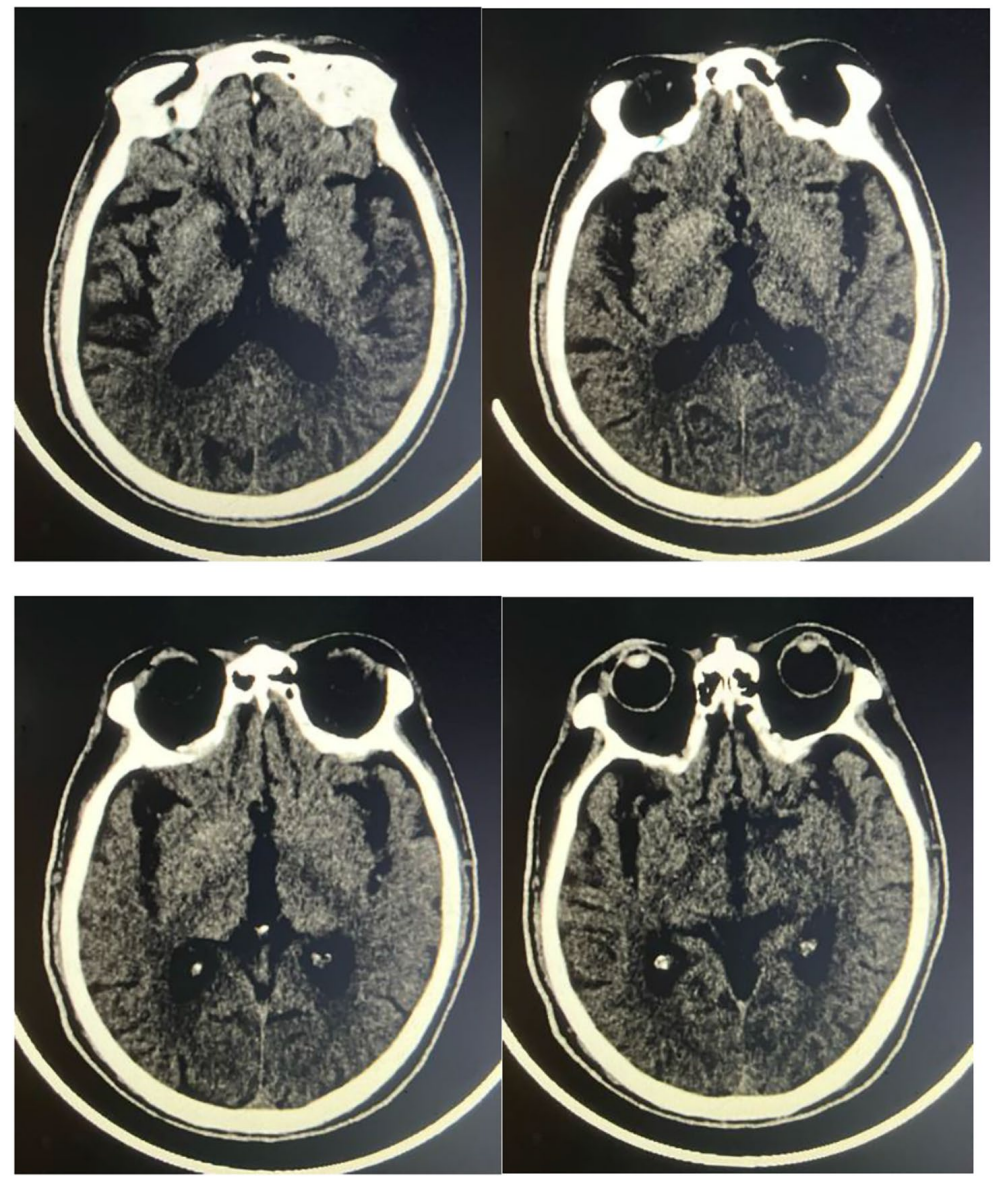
The patient’s imaging results. Brain CT showed a significant decrease in CT values of high-density lesions in both basal ganglia regions compared to previous studies
